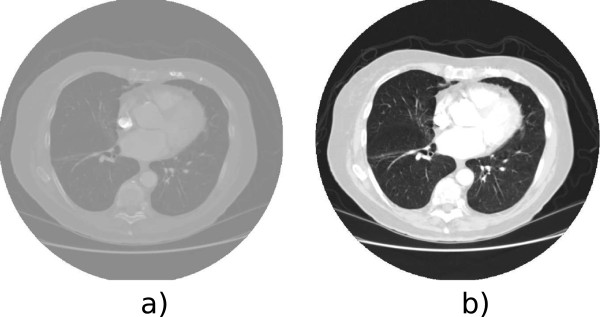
Preprocessing of a chest CT scan. a) original image b) image with changes on opacity, color and gradient. Removing defects caused by lack of contrast in the image acquisition process by filters of opacity, color and gradient to improve the image quality.
